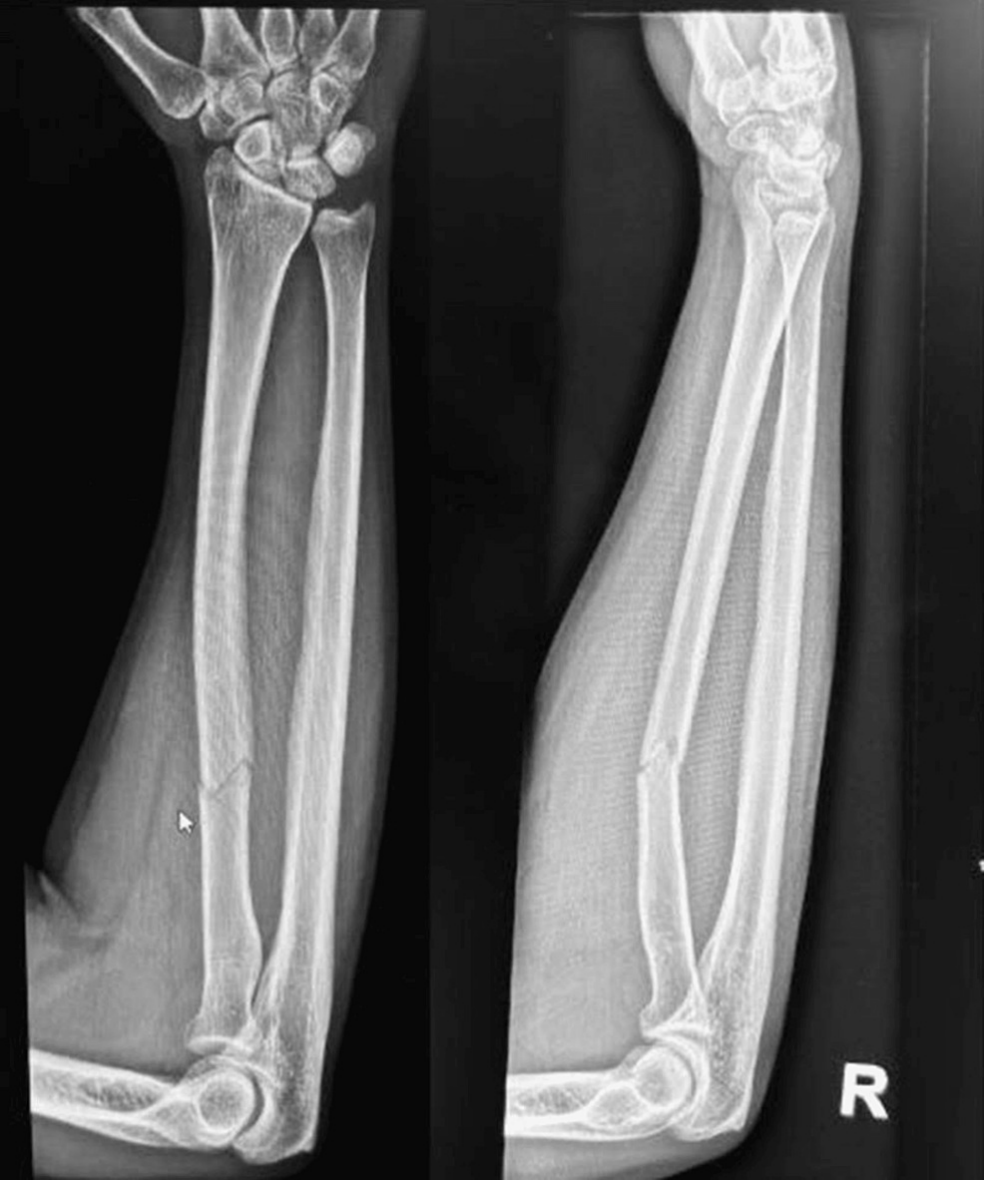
Preoperative AP and lateral view X-rays of the right forearm demonstrating proximal radial shaft fracture
